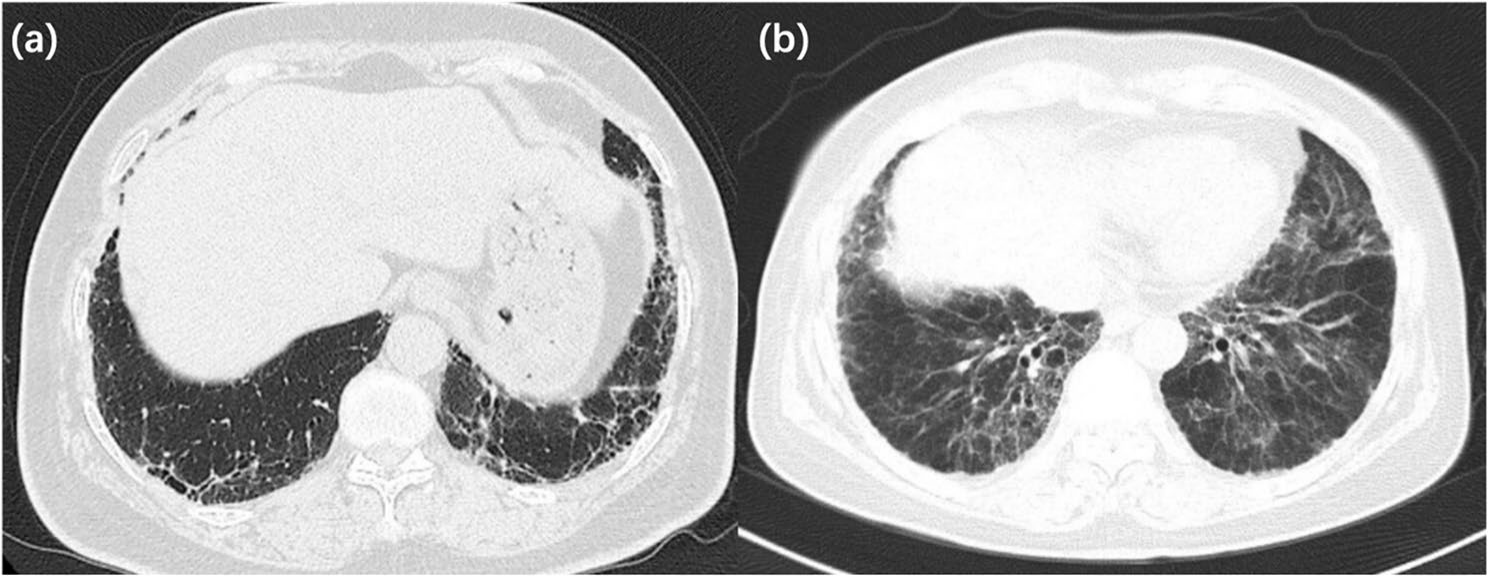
The typical UIP pattern (a) and NSIP patten (b) on chest CT pattern in this study
